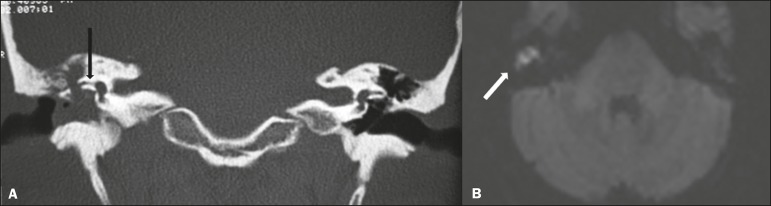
Cholesteatoma. A: Coronal CT, with a bone window, showing
